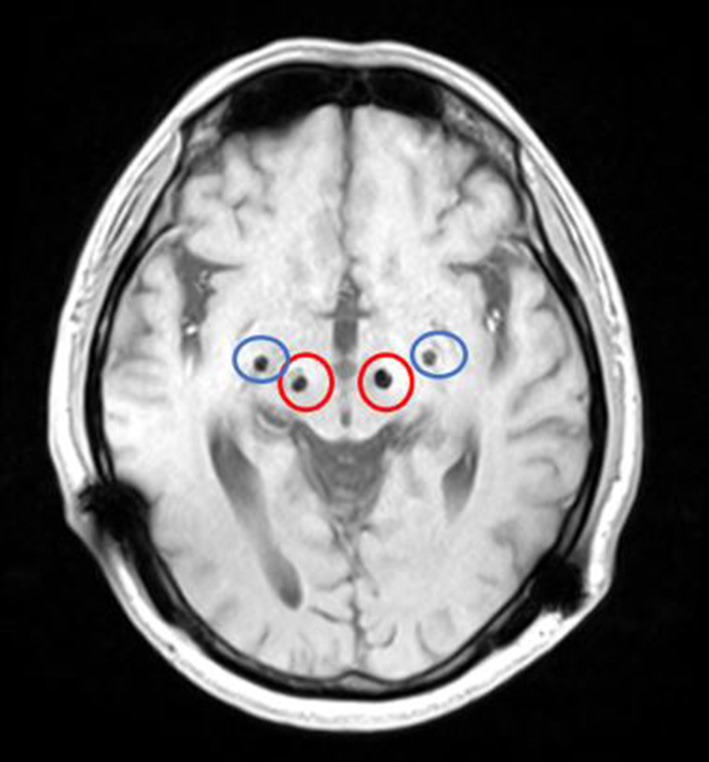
MRI (magnetic resonance imaging) after bSCG-DBS implantation (the red circle is STN, and the blue circle is GPi).
